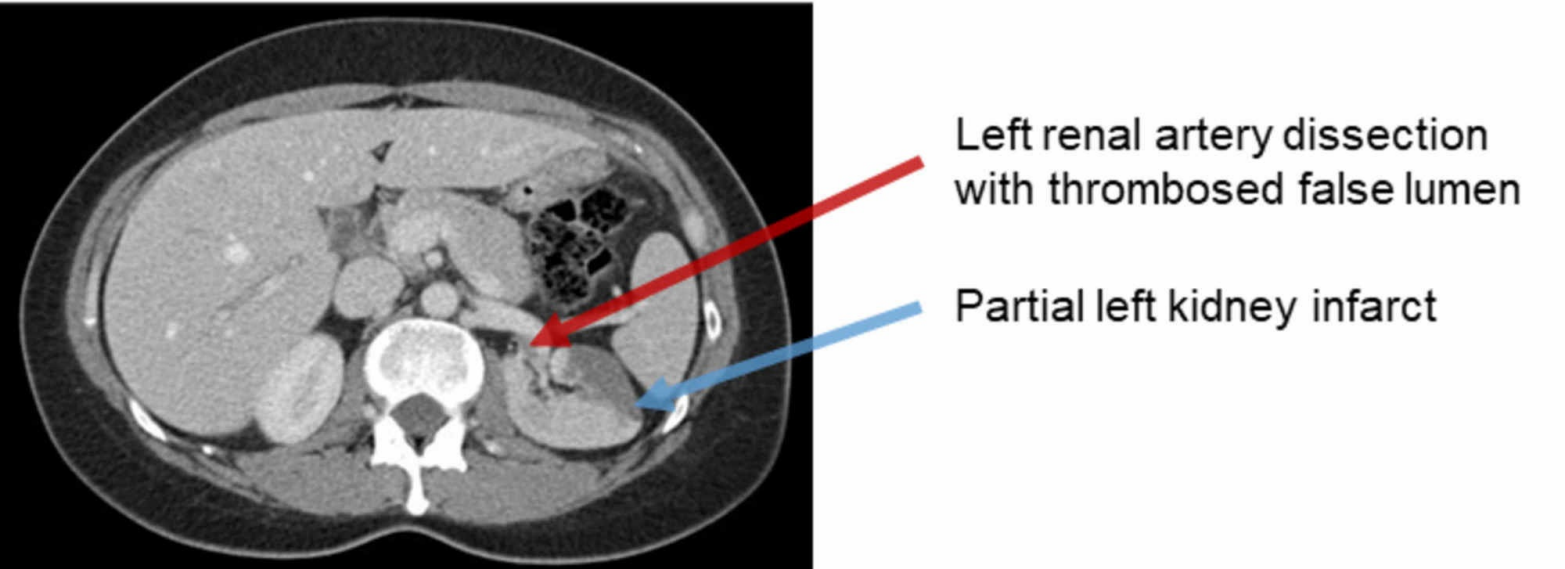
CT abdomen and pelvis: left renal artery dissection with a thrombosed false lumen and partial left kidney infarct CT, computed tomography
